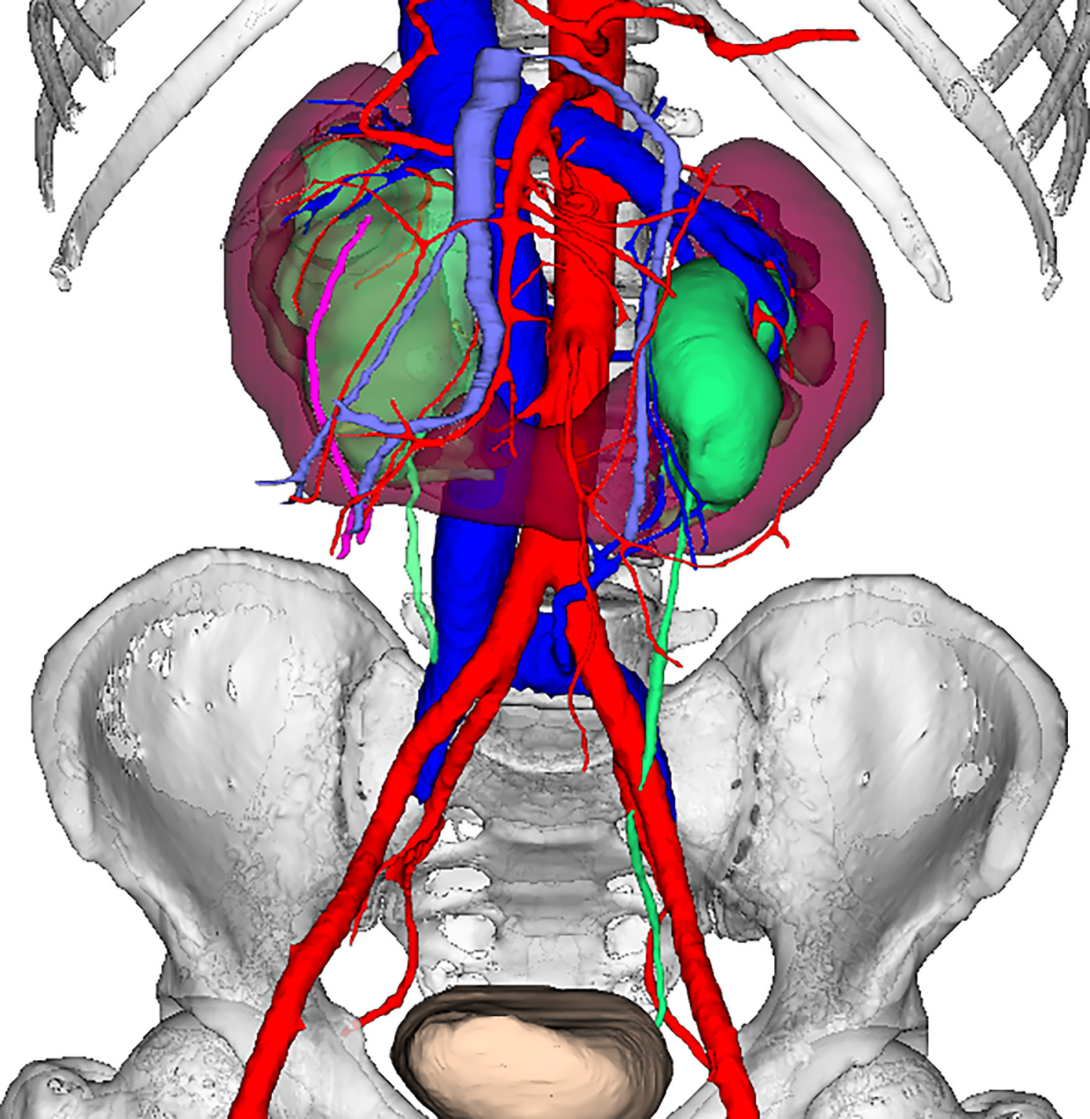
The three-dimensional image reconstructed by urinary enhanced computed tomography of the patient.
